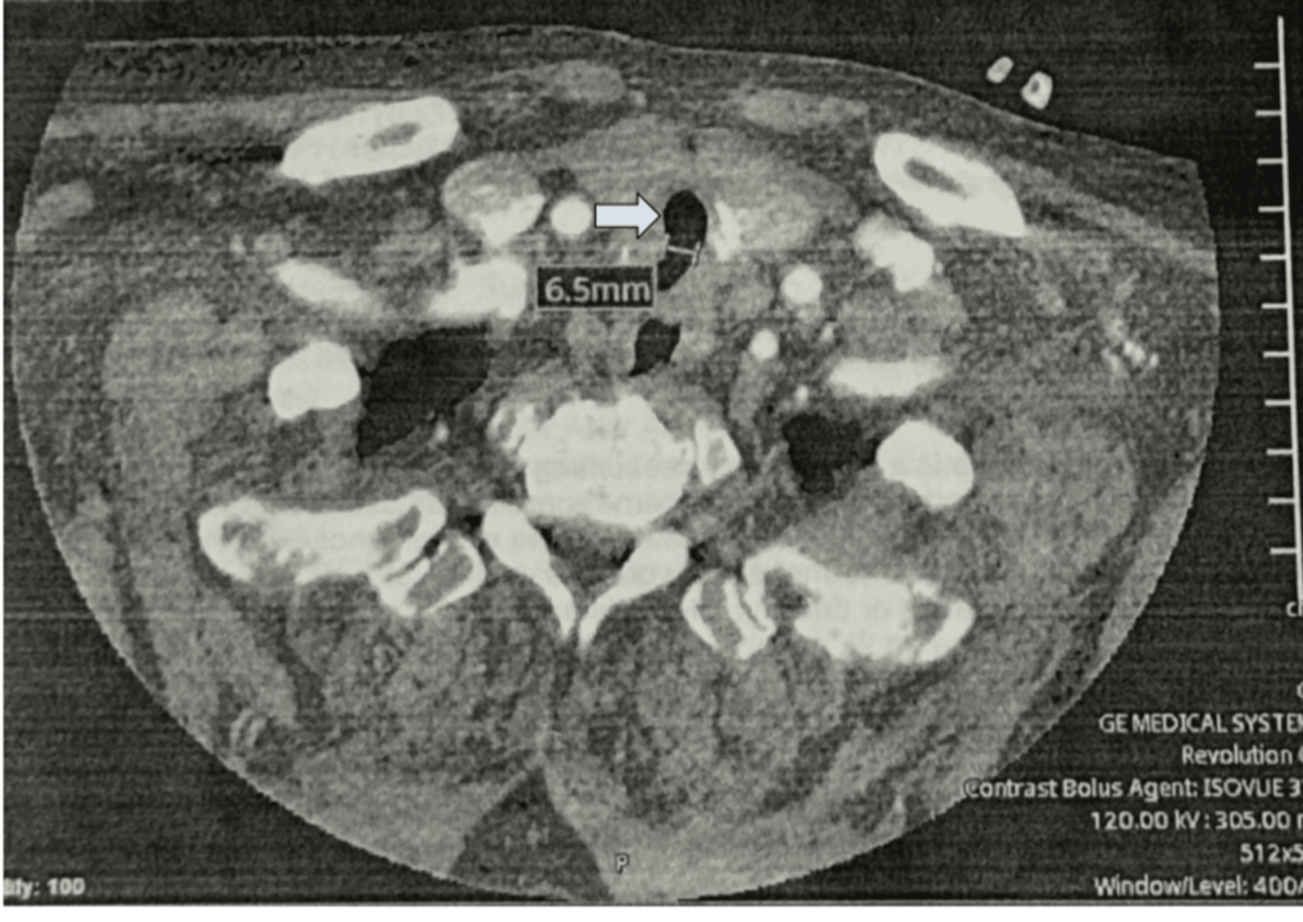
CTA of the patient's head and neck from 2024 revealing subglottic stenosis CTA: computed tomography angiography
